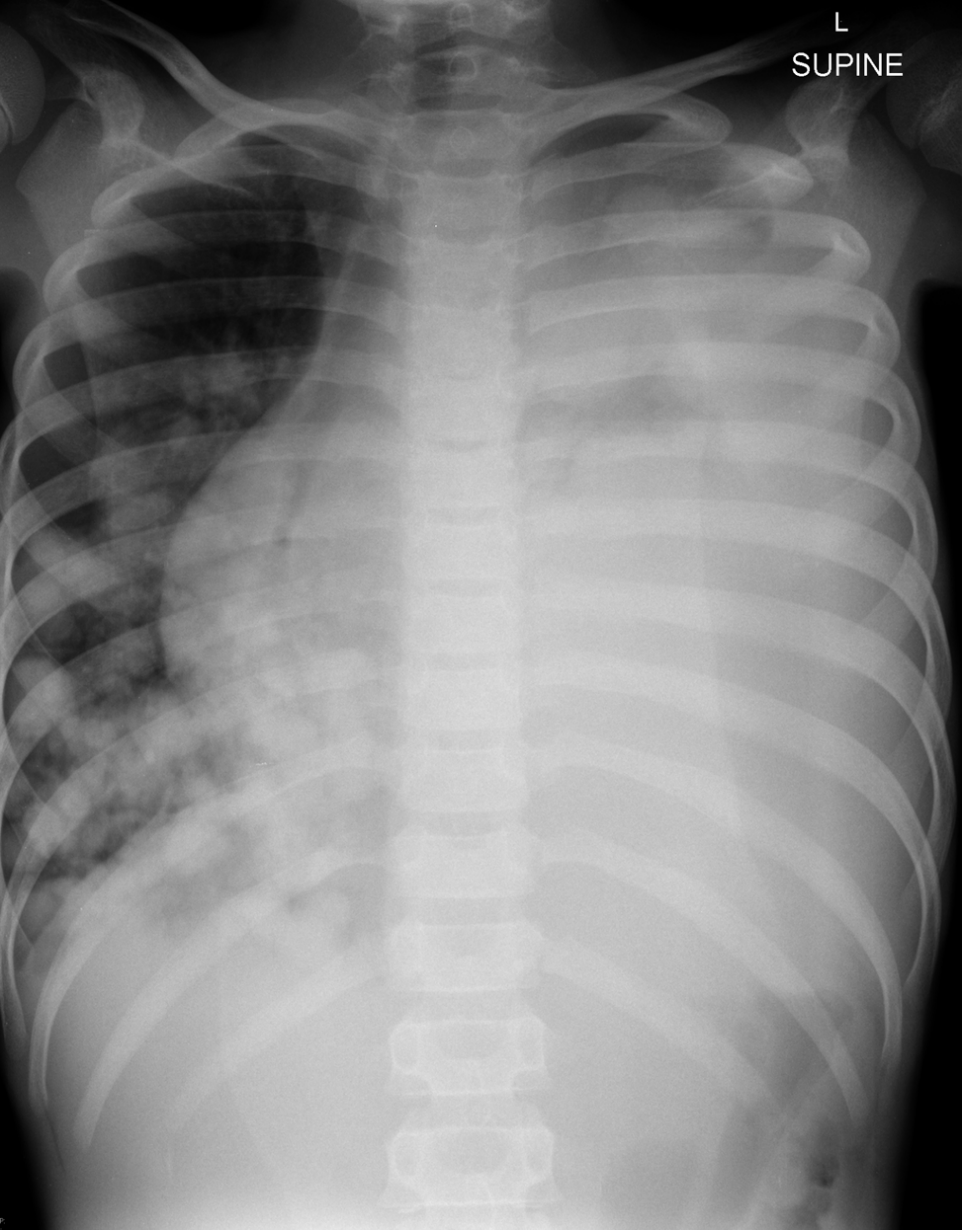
Chest x-ray of a 10-year-old patient presenting with respiratory distress, productive cough, and pleuritic chest pain shows complete opacification of the left lung and multiple round opacities in the right middle and lower lung fields.
